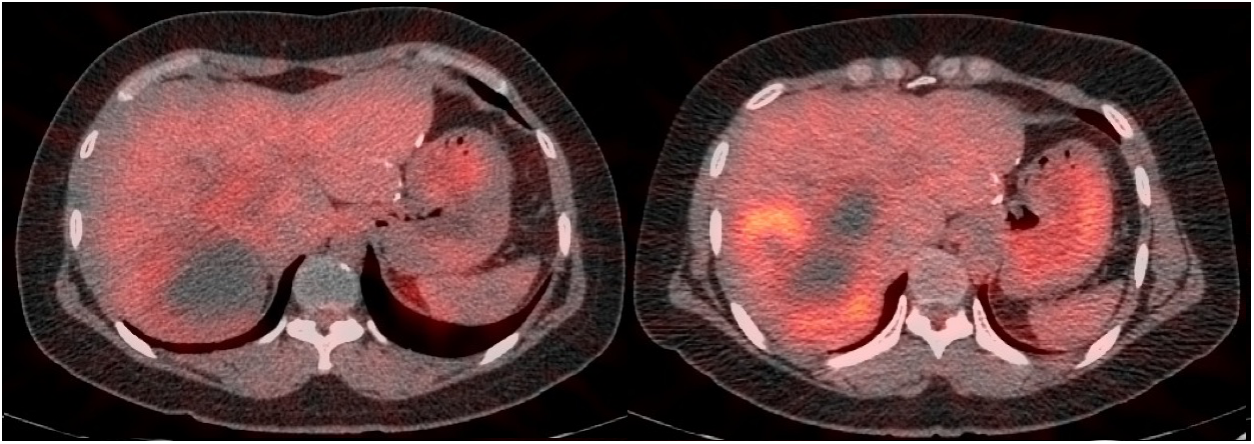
Fusion PET/CT scans at baseline (A.Pre) and after 4 weeks of therapy with pazopanib in a patient with well-differentiated thyroid. Post-pazopanib scan (B.Post) demonstrates new disease along superomedial margin of RFA site (Patient 2). RFA, Radiofrequency ablation; PET, positron-emission tomography.
